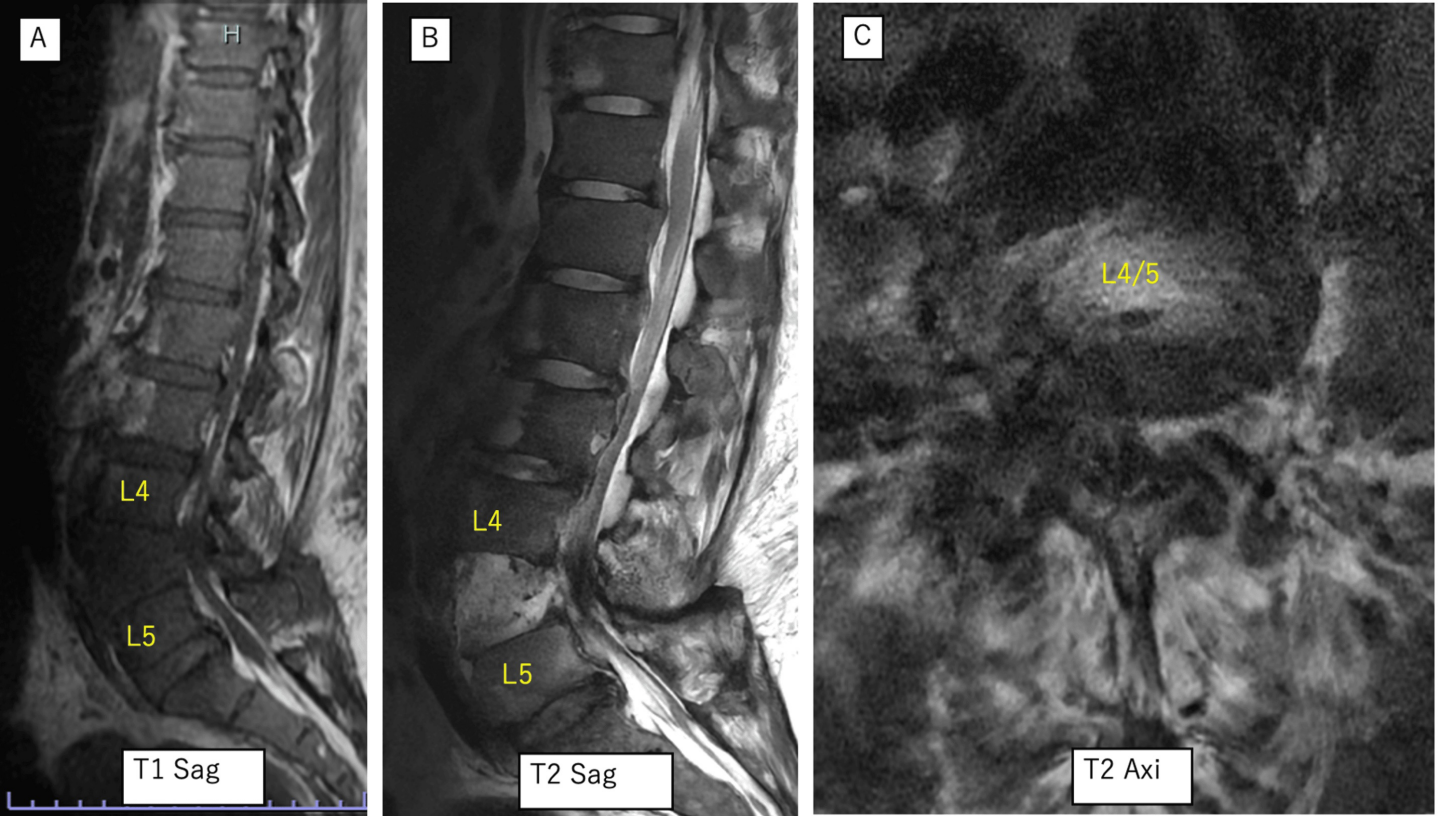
Preoperative magnetic resonance imaging demonstrating cauda equina compression at L4/L5. (A) Sagittal T1-weighted image; (B) sagittal T2-weighted image; (C) axial T2-weighted image showing severe compression of the cauda equina at the L4/L5 level.
